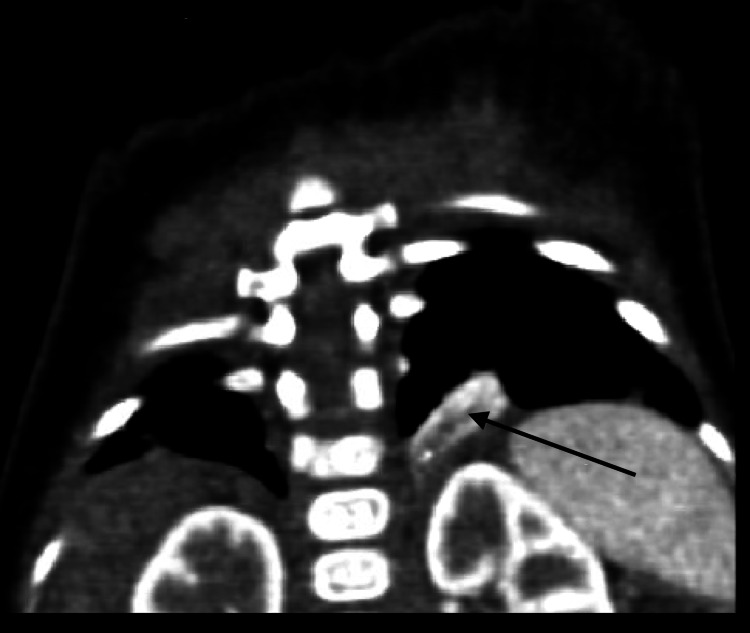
Coronal CT view showing extralobar pulmonary sequestration (black arrow)
